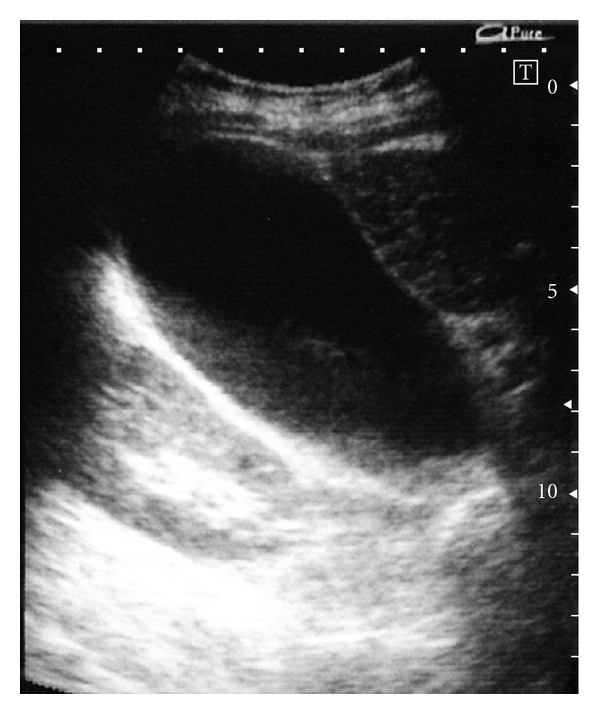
Clear thickening of the gallbladder wall cannot be seen on abdominal ultrasonography, but echoes from biliary debris can be seen inside a highly tense and enlarged gallbladder. Clear elevated lesions and calculi cannot be seen in the gallbladder.
